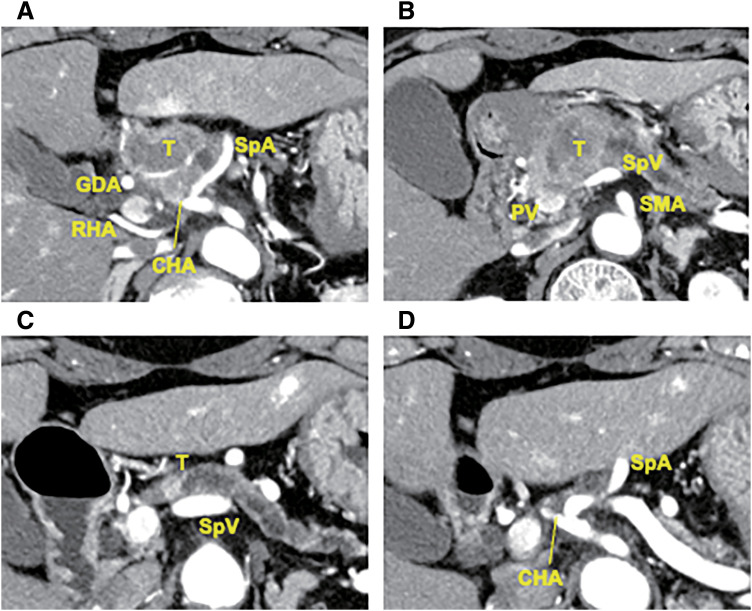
Contrast-enhanced abdominal CT scan before (A, B) and after neoadjuvant chemotherapy (C, D). (A) A heterogeneously enhanced tumor measuring 32 mm was observed in the pancreatic head region, with possible involvement of the common hepatic artery over 180°. (B) The root of the splenic vein was invaded. (C) The tumor size was significantly reduced to 8 mm. (D) The tumor was no longer in contact with the arteries. CHA, common hepatic artery; CT, computed tomography; GDA, gastroduodenal artery; PV, portal vein; RHA, right hepatic artery; SMA, superior mesenteric artery; SpA, splenic artery; SpV, splenic vein; T, tumor
